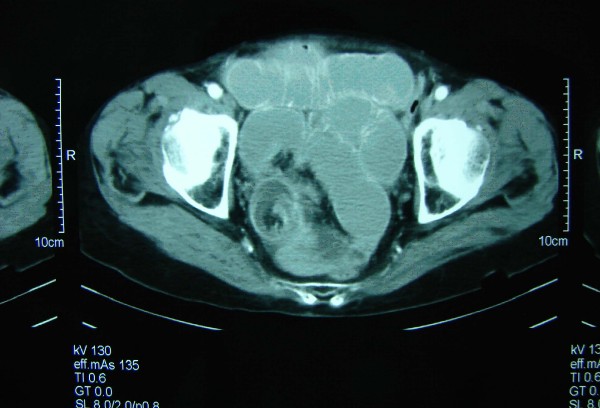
Abdominal computed tomography scan showing the characteristic "target sign", establishing the diagnosis of intussusception.
